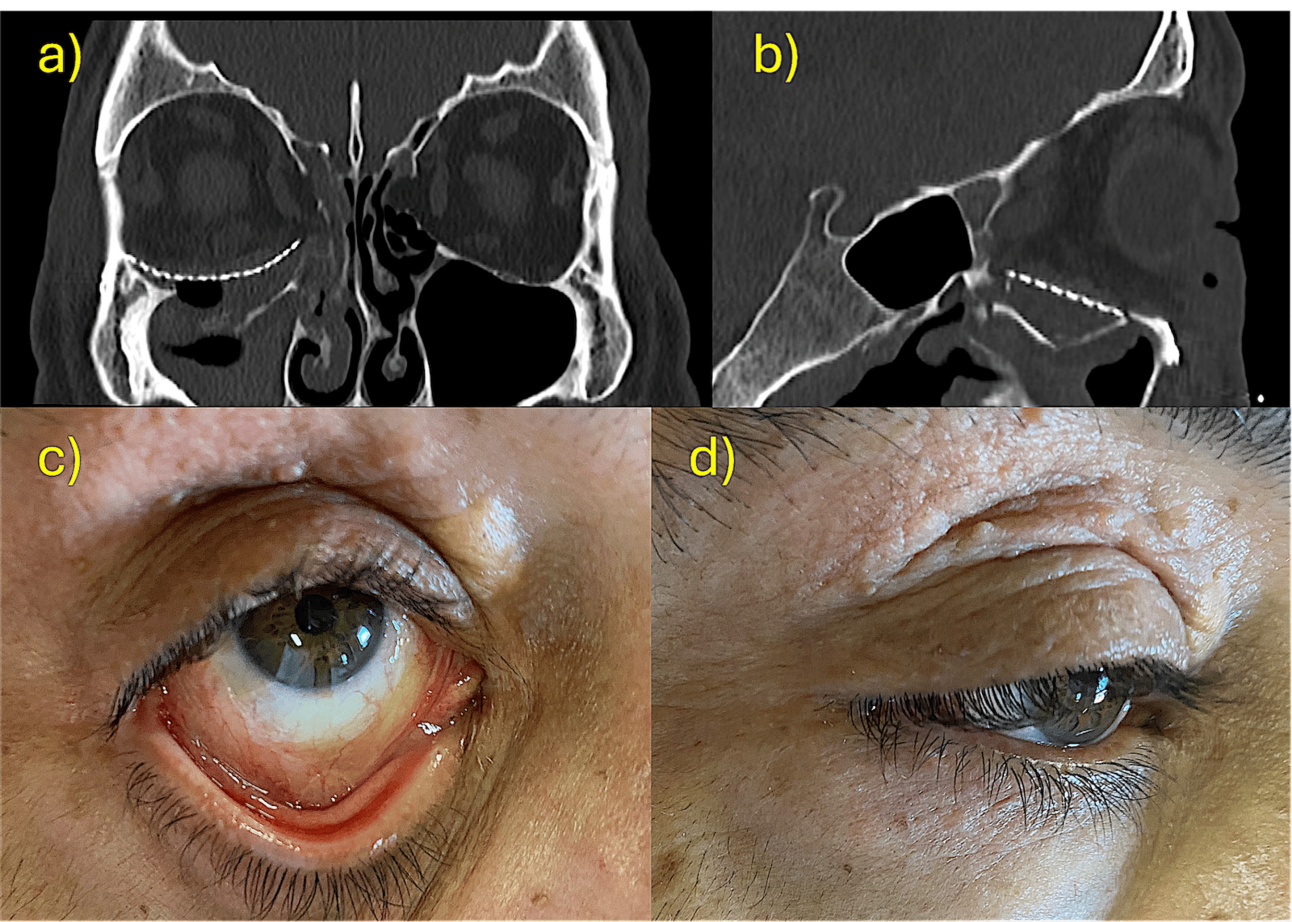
Postoperative results (a) CT scan at 24 hours postoperative (coronal view). (b) CT scan at 24 hours postoperative (sagittal view). (c) Favorable transconjunctival access scar (one month postoperative). (d) Inconspicuous lateral canthotomy extension scar (one month postoperative).
